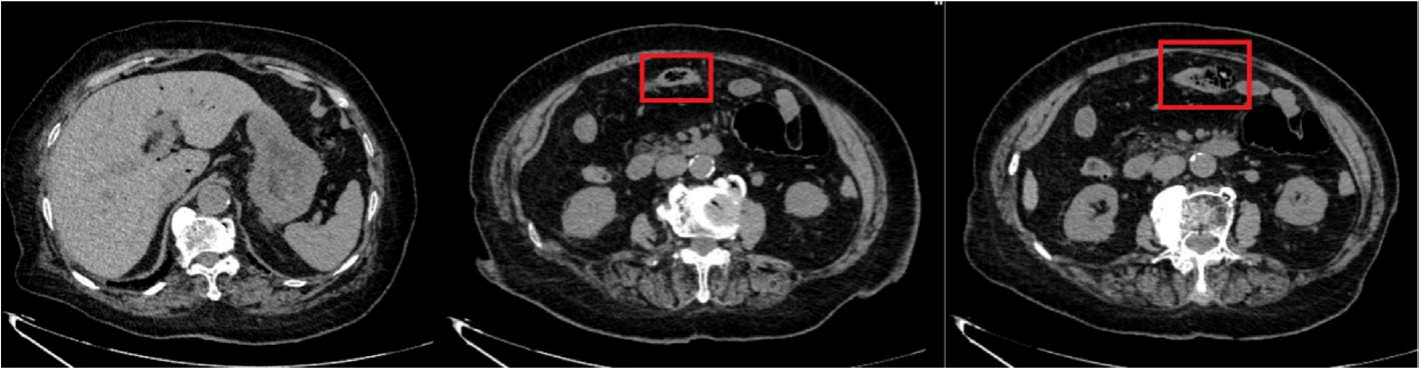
Initial Non-Contrast Abdominal CT (External Institution, November 17, 2024)
